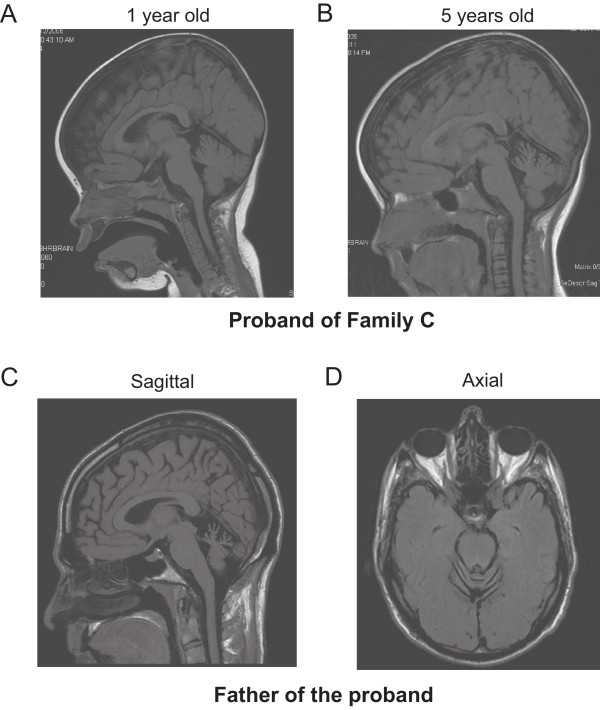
Neuroimaging features of Family C. A. T1 weighted sagittal MRI of the brain of the proband from Family C demonstrating mild cerebellar hypoplasia at 1 year of age. B. Demonstration of cerebellar atrophy in the proband at 5 years of age. C. and D. T1 weighted sagittal and axial MRI of the brain of the proband’s father at 45 years of age demonstrating diffuse cerebellar atrophy.
